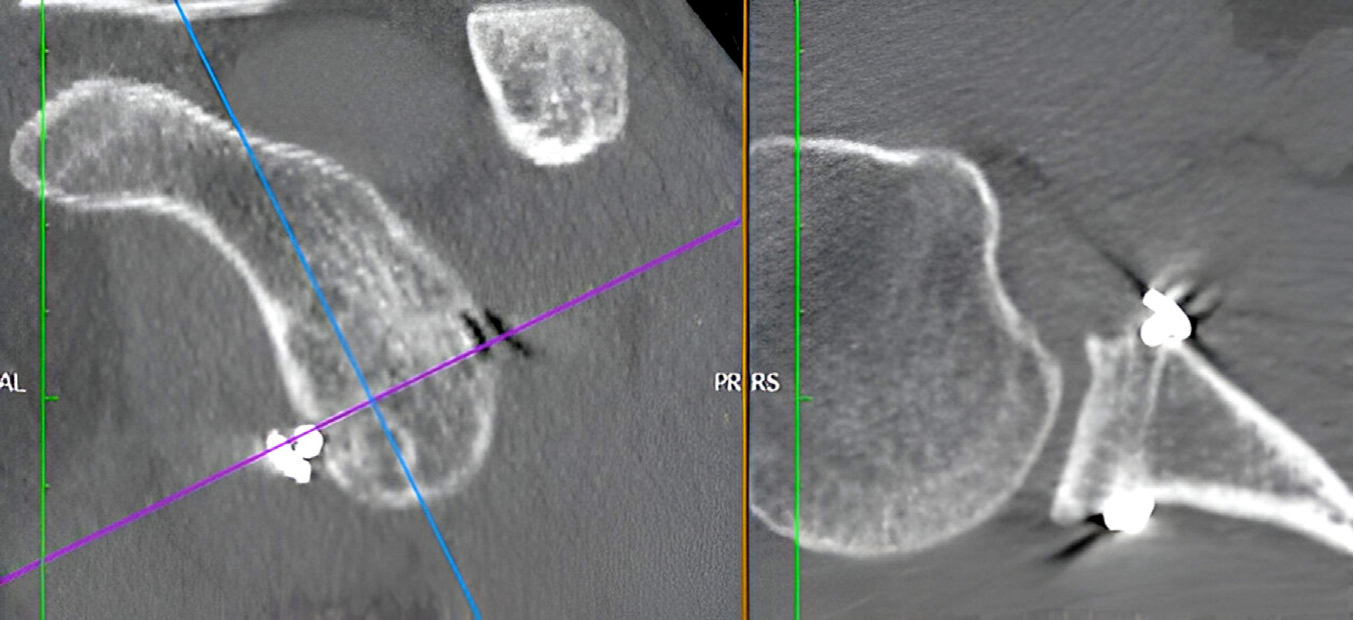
Last follow-up CT scan evaluation. Showing complete bone graft resorption and poor glenoid reconstruction. *CT*, computed tomography.
